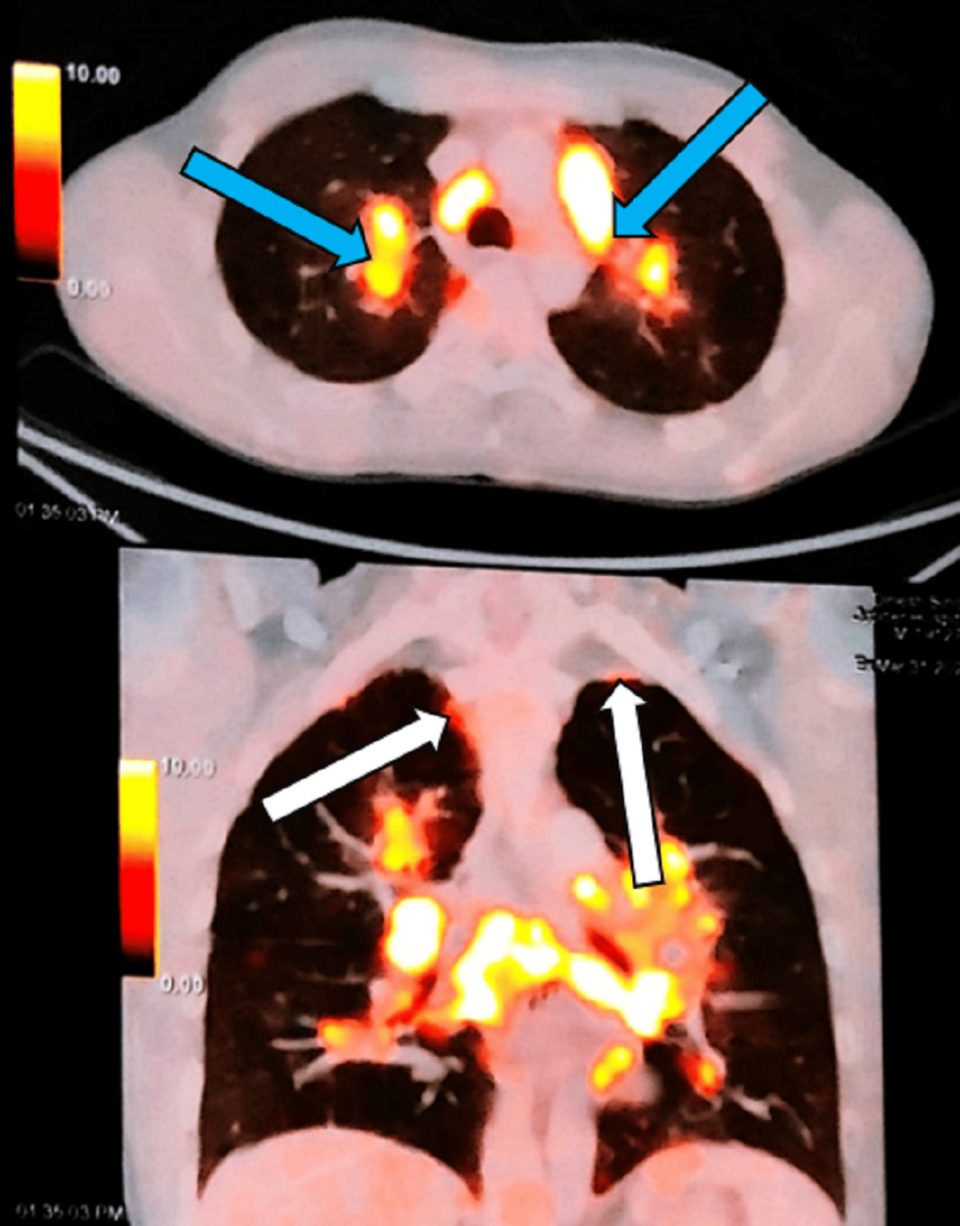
PET-CT scan showing metabolically active, multiple mediastinal, and bilateral hilar lymphadenopathy (blue arrows) and metabolically active bilateral pleural nodules (white arrows). PET-CT: positron emission tomography-computed tomography
